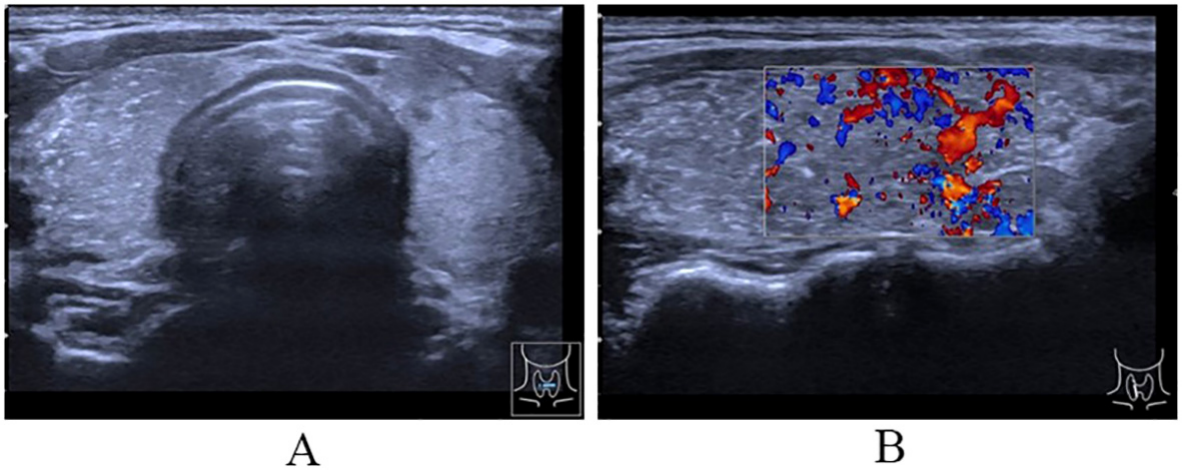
Ultrasound of thyroid. (A) The cross-section showing uneven internal echo of the thyroid gland with scattered bright spots; (B) The longitudinal section showing diffuse sclerosis of the thyroid gland and abundant blood flow signal in thyroid parenchyma.
